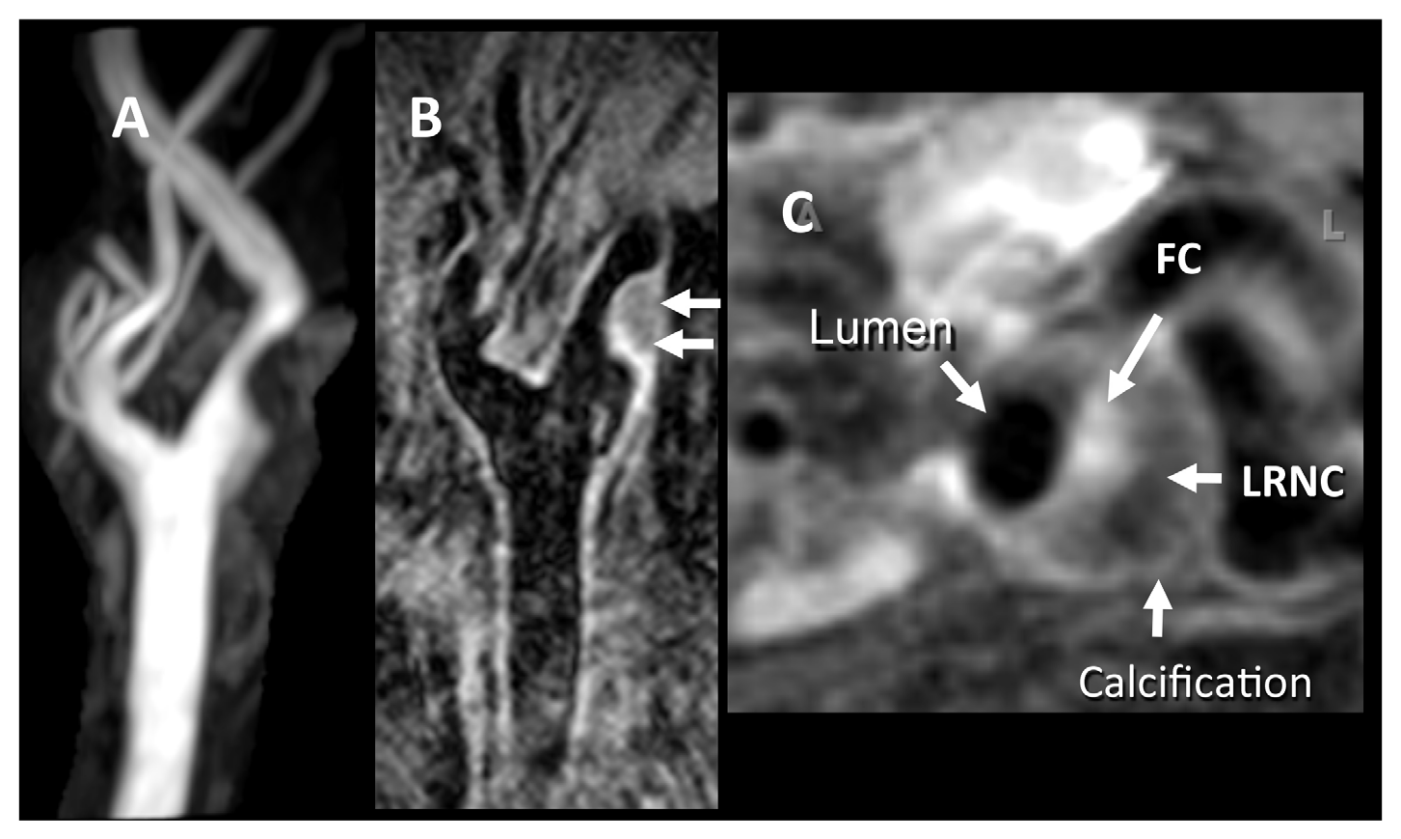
Features of complex plaque on magnetic resonance imaging. (
